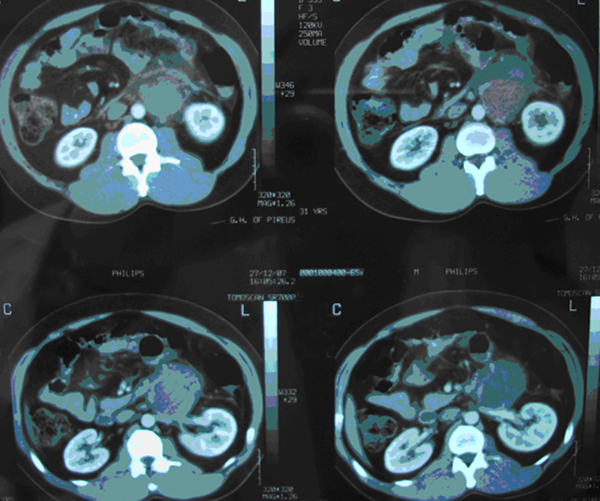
Computed tomography scan showing a 7 cm retroperitoneal tumor on the left side of the aorta, compressing the left renal vein.
